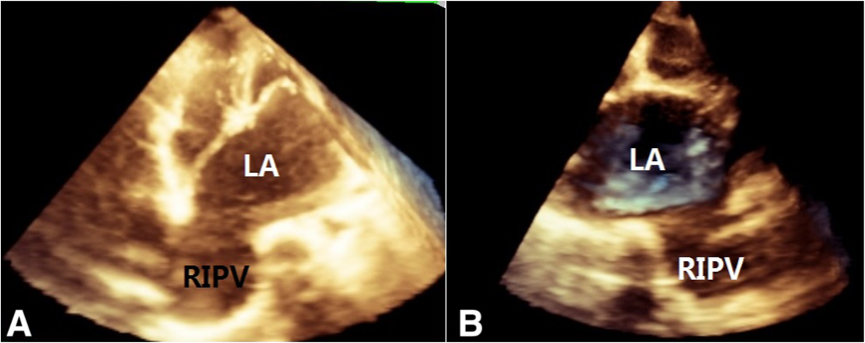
Three-dimensional echocardiography showing a clear stereoscopic view of the right pulmonary venous aneurysm. (A) The three-dimensional echocardiographic view in apical view reveals the enlarged LA and RIPV. (B) The three-dimensional echocardiographic view in parasternal view shows the large LA and RIPV. LA, left atrium; RIPV, right inferior pulmonary vein.
